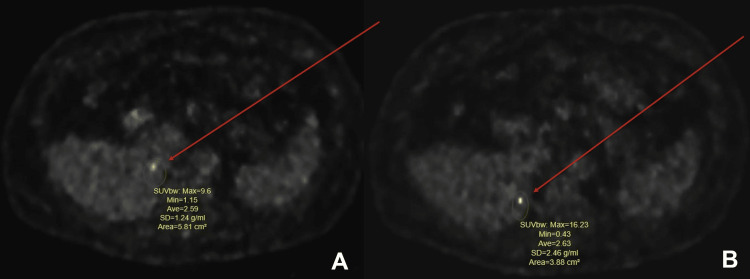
Ga-68 PET-CT with images of two hypermetabolic foci in the medial aspect of the lower right lobe of the liver, representing metastatic liver lesions
